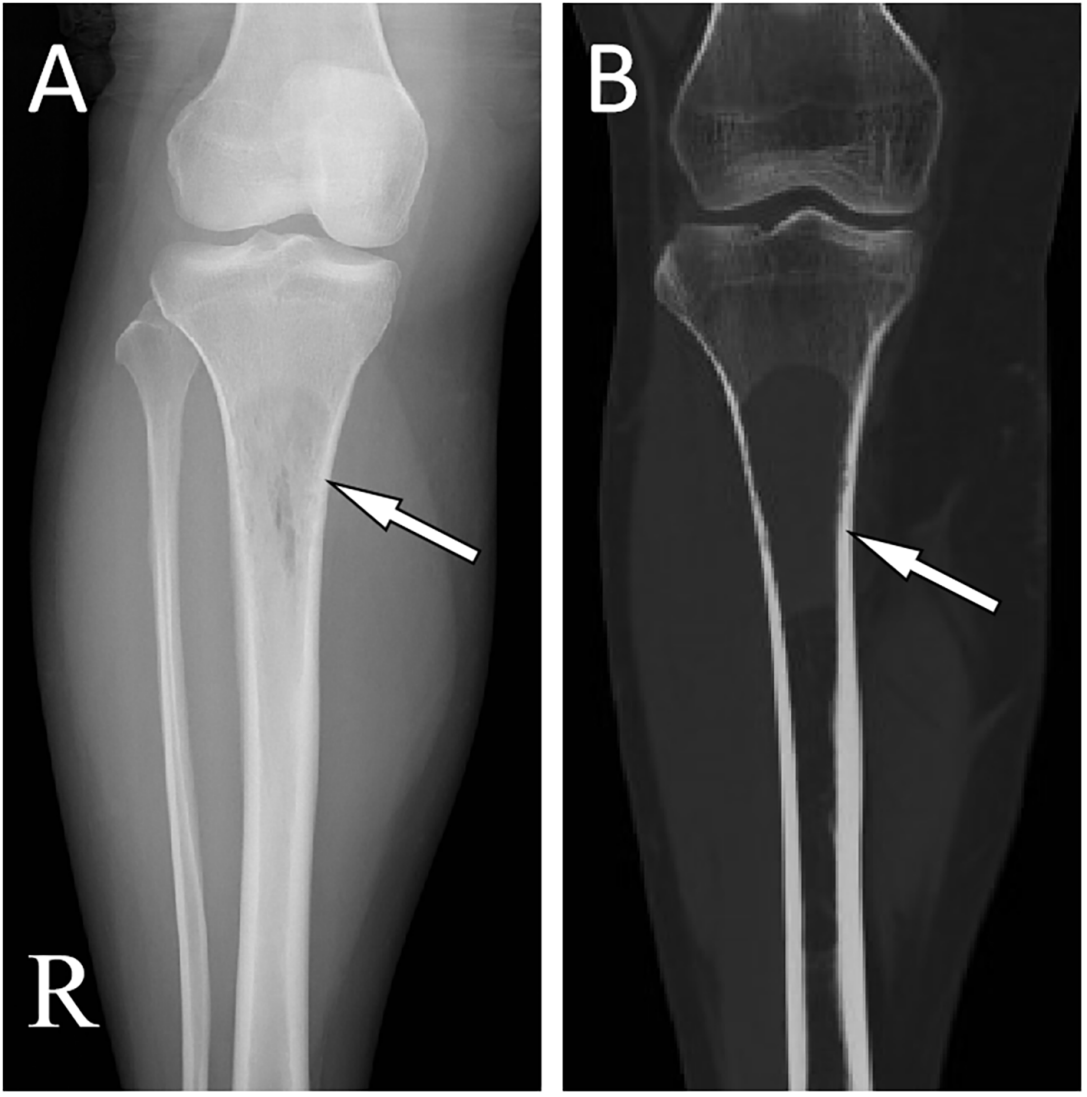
(A) X-ray shows a low-density shadow along the longitudinal axis at the proximal end of the right tibia without periosteal reaction and sclerotic rims (arrow). (B) CT showed that the normal trabecular structure of the proximal end of tibia disappeared, the cortical bone was intact, and no obvious soft tissue mass was found (arrow).
